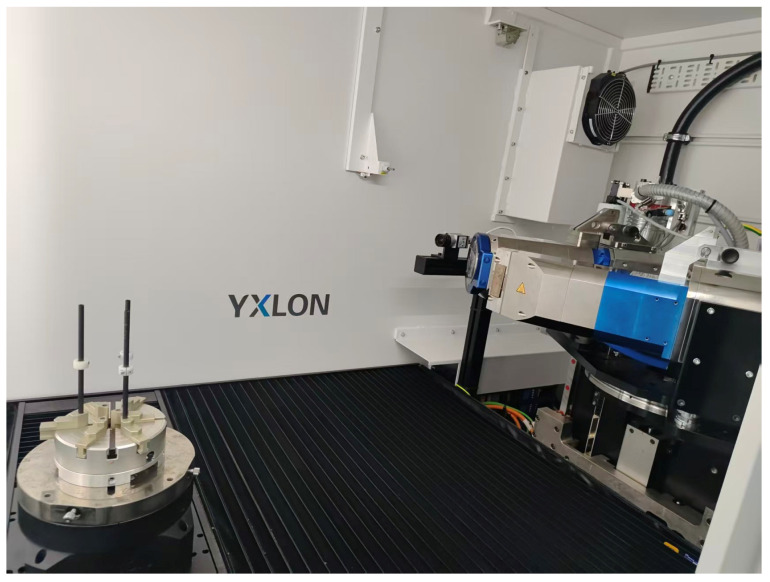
The CTYXLON FF35 industrial CT.
